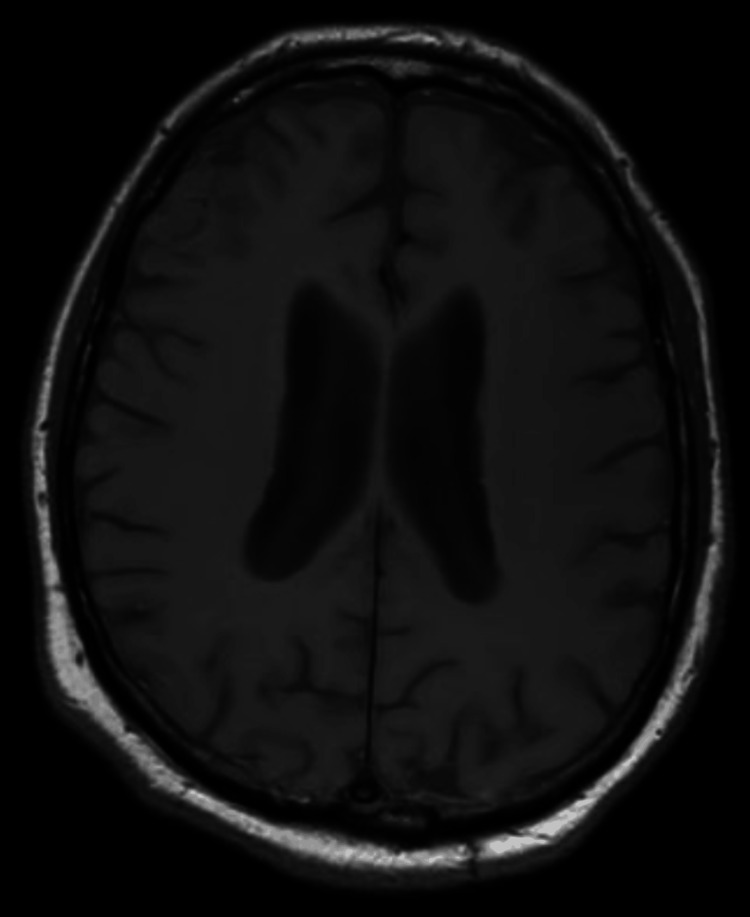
Brain MRI (T1 axillar) without contrast showed enlarged lateral ventricles.
